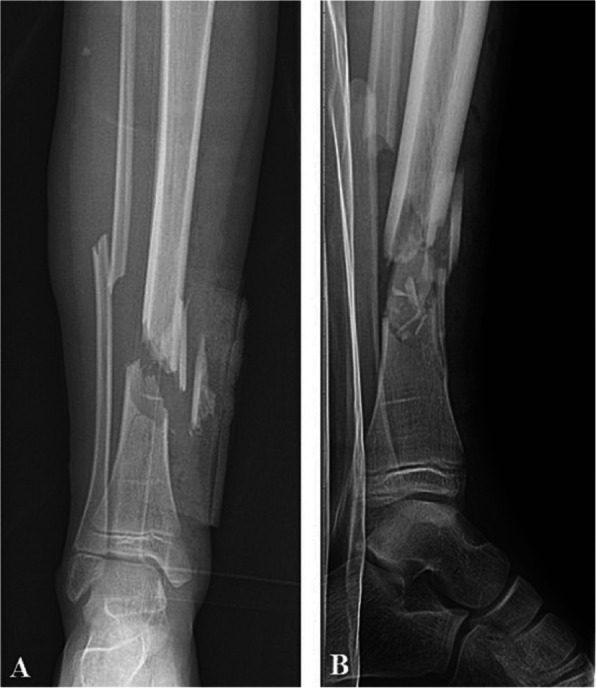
Example of comminuted fracture pattern of tibia fracture X-ray images in (a) anterior–posterior and (b) lateral view [13]
